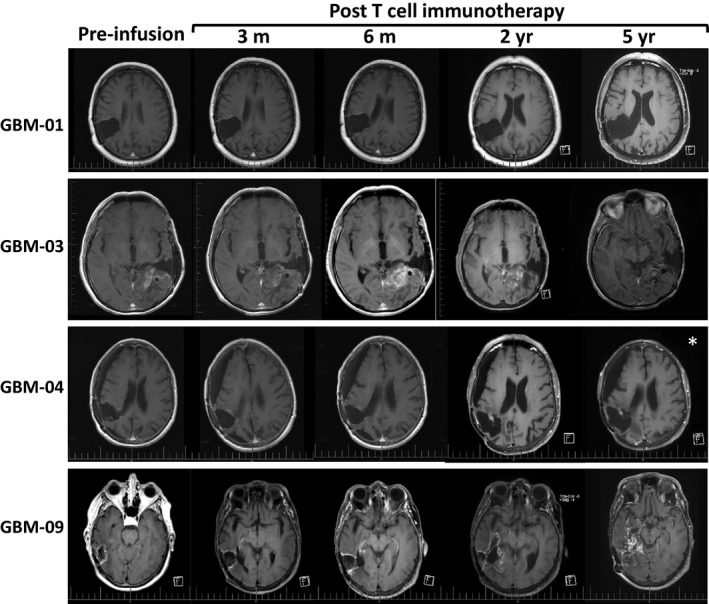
Representative T1^+^Gad axial MRI scans for long‐term survivors. In patient GBM‐03, enhancing tissue in the tumor bed became progressively more prominent over time, but there was little mass effect associated with this and the patient was clinically stable. Our interpretation therefore was that this enhancing tissue did not represent recurrent tumor. GBM‐04 showed no evidence of recurrent disease until the time of her untimely death 2.5 years after T‐cell infusion (*) from a basilar artery aneurysm (unrelated to adoptive T‐cell therapy).
